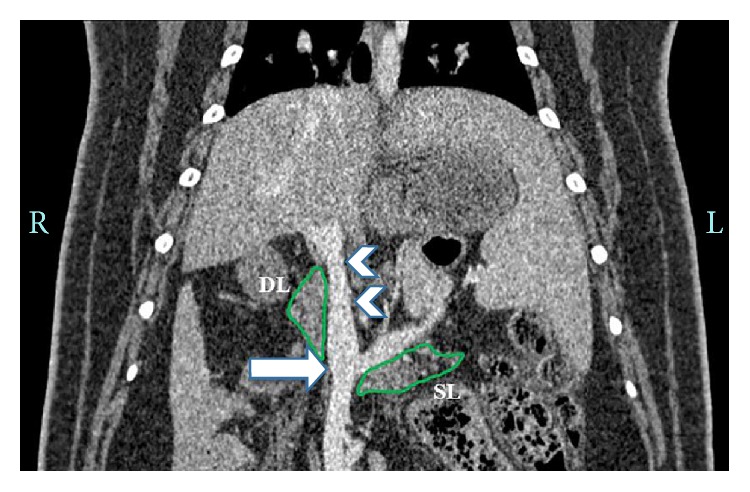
Preinterventional planning CT angiography. Coronal CT angiogram demonstrating the normal vascular anatomy of the portal venous system. Note the splenoportal confluence (arrow) and the long extra-hepatic course of the portal vein (arrowheads) in relation to the pancreatic duodenal lobe (DL) and the splenic lobe (SL). Both pancreas lobes are outlined in green.
